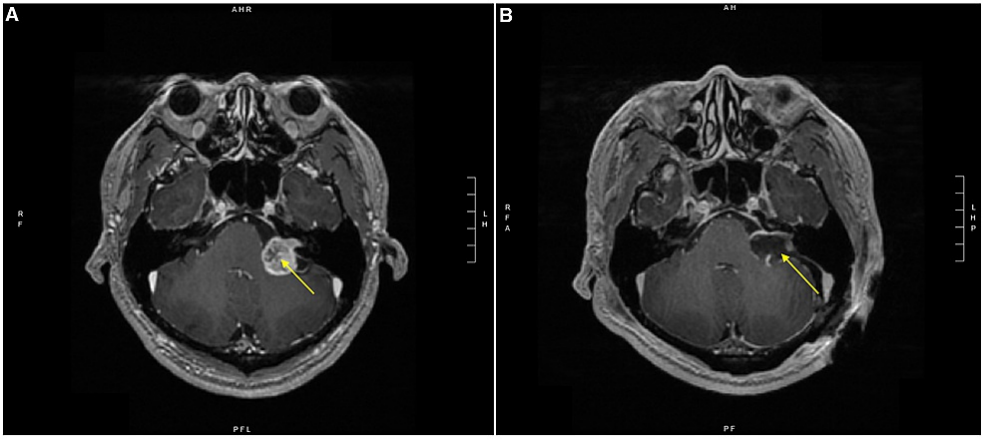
T1-weighted brain MRI. (A) Preoperative brain MRI with a yellow arrow pointing to the vestibular schwannoma in the left cerebellopontine angle. (B) Postoperative brain MRI with a yellow arrow pointing to postsurgical changes following debulking of the left vestibular schwannoma and a residual ring of enhancing tumor. MRI: magnetic resonance imaging
